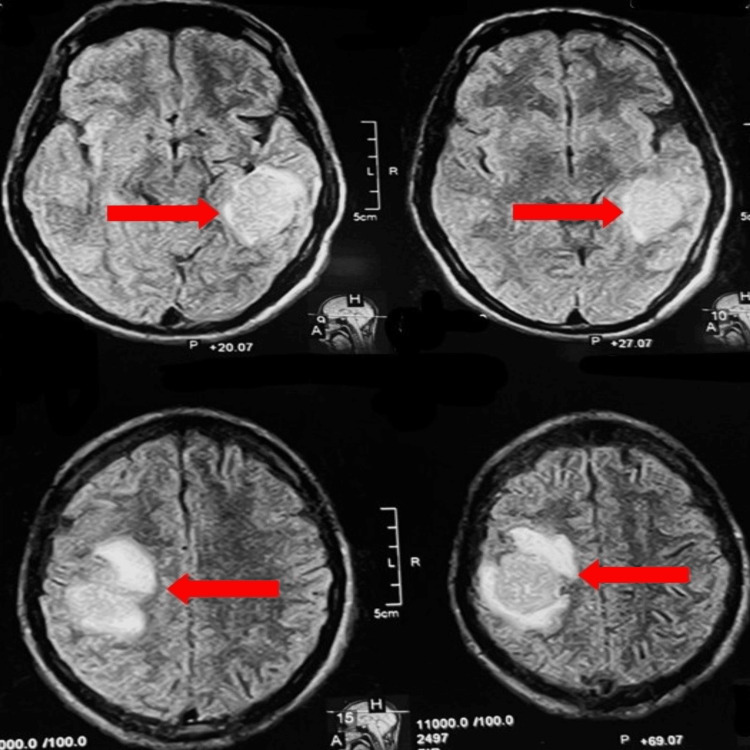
Contrast-enhanced magnetic resonance imaging of the brain (axial view) showing bilateral space-occupying lesions indicating metastases (red arrows)
